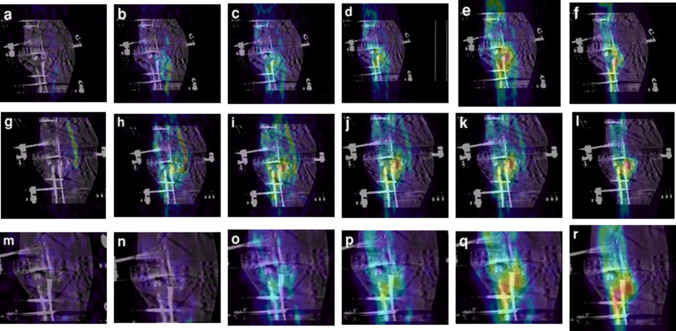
A sagittal slice from the CT scan at the level of the crural fraction for Patient 15 is shown superimposed on the corresponding PET slice. The columns represent the radionuclide uptake at the times 30 s, 40 s, 60 s, 120 s, 180 s, and 45 m after the initial injection. The first scan is shown in (a–f). After the first revision, the second scan is seen in (g–l) and the third scan after ultrasound stimulation is shown in (m–r). The radionuclide uptake is more uniform across the crural fracture in (m–r)
